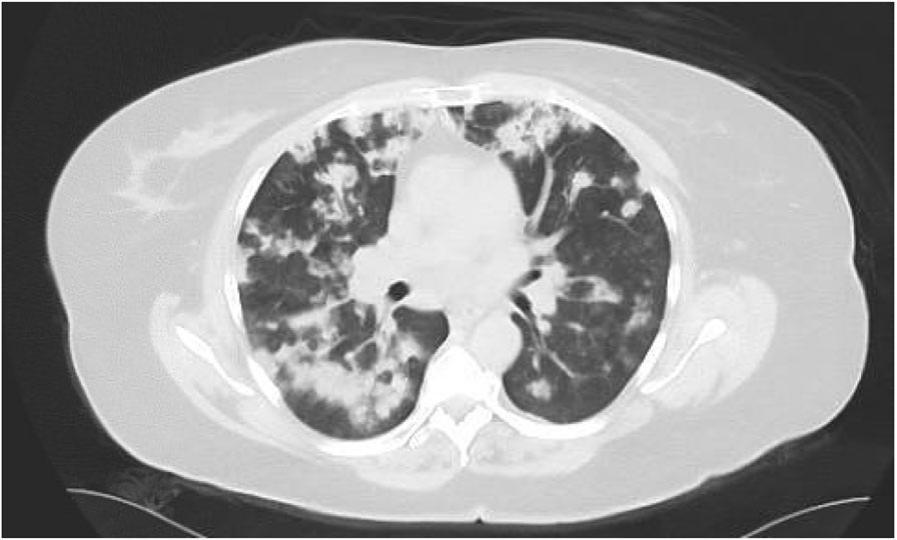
Chest computed tomography shows bilateral irregularly shaped nodules asymmetric on the right with peripheral and pleural-based distribution
